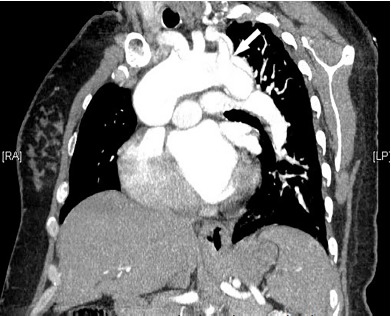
Computed tomography thoracic aorta showing aortic dissection involving left common carotid artery (arrow).
